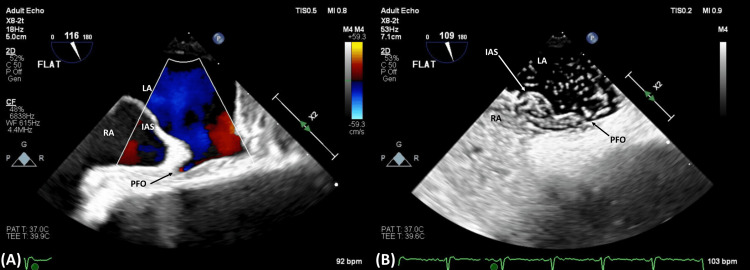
Trans-esophageal echocardiogram (TEE) while laying flat, demonstrating mild right-to-left shunting via PFO – (A) Doppler study; (B) agitated saline/bubble study RA = Right Atrium; LA = Left Atrium; IAS = Interatrial Septum; PFO = Patent Foramen Ovale
